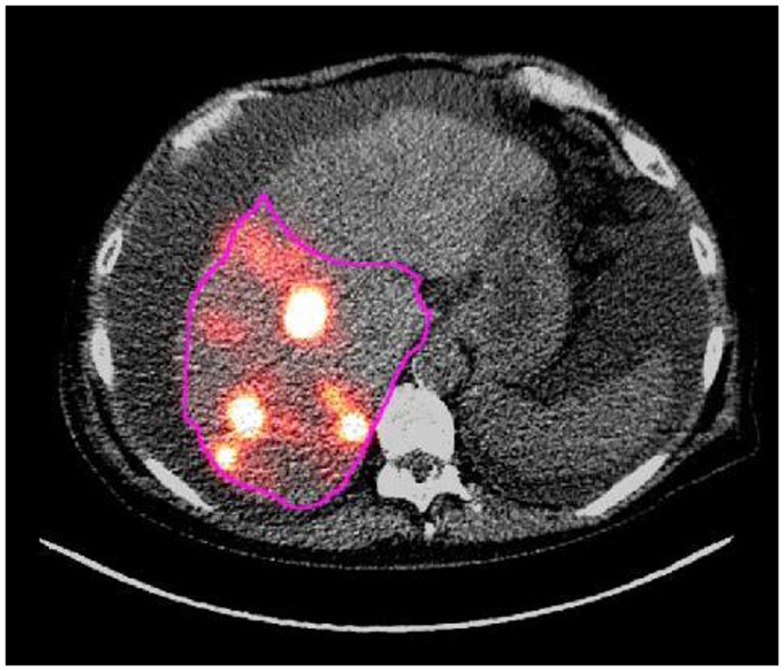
This image shows a contour in pink drawn around a treated region in an axial slice of the AC CT/PET fusion image that was acquired following right lobe ^90^Y microsphere therapy.
